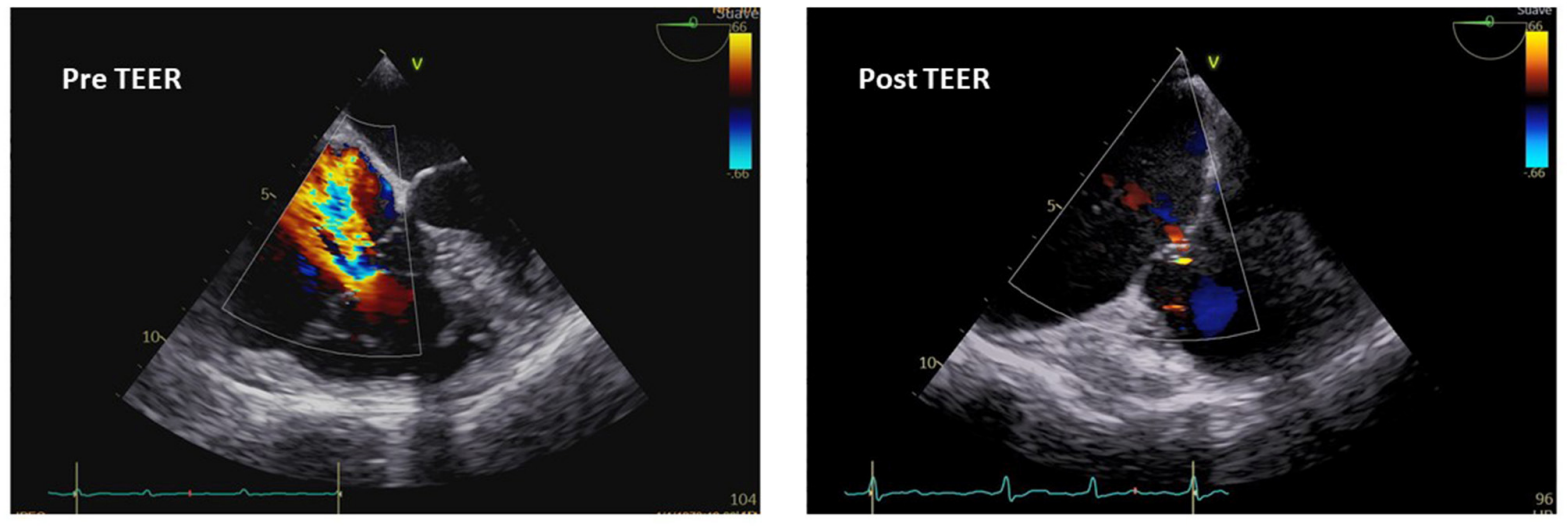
Transesophageal echocardiography imaging and pre- and postoperative assessment of TR after transcatheter edge-to-edge repair (TEER).
